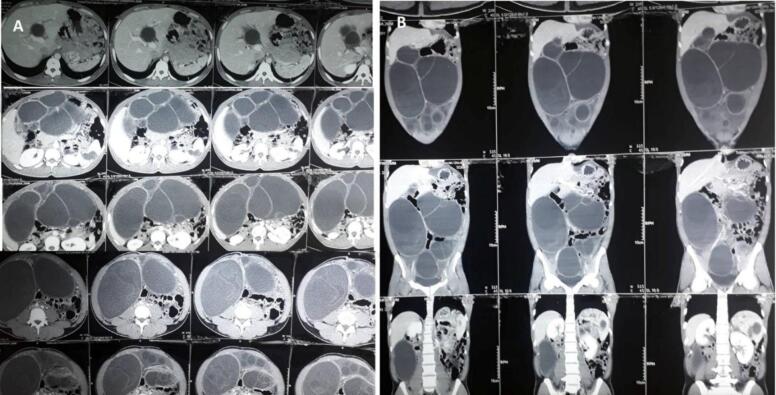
Computed tomography scan of abdomen and pelvis (A: axial, B: coronal section) revealing multiple cystic masses extending throughout the abdomen.
